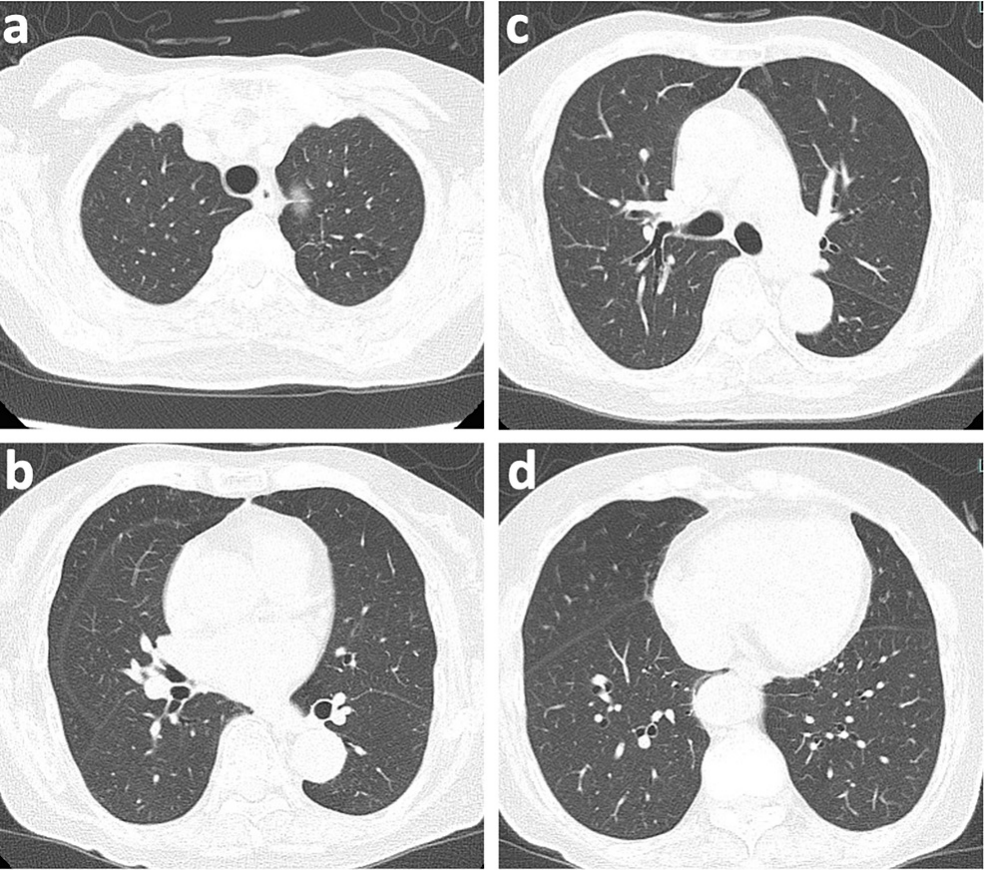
(a-d) Chest CT images of the patient on day 19 showing complete resolution of the ground-glass opacities
